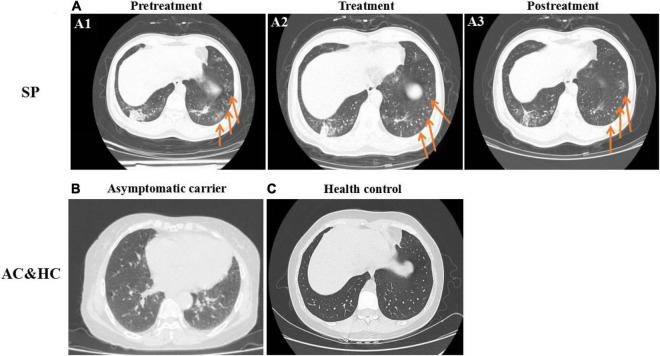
Typical chest CT imaging in SPs, asymptomatic carriers (ACs), and healthy controls (HCs) (same abbreviations in subsequent figures). (A) CT imaging in SPs. (A1) Pretreatment. (A2) Treatment. (A3) Posttreatment. (B) CT imaging in ACs. (C) CT imaging in HCs. Bilateral pneumonia and multiple ground glass shadows were obvious in SPs and were marked with arrows (A1–A3), whereas pulmonary lesions in ACs (B) were not obvious compared with the control (C).
